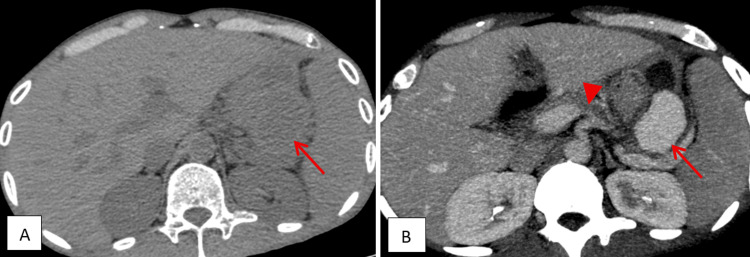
Axial sections of the non-enhanced (A) and venous phase (B) contrast-enhanced CT abdomen show a splenic vein varix within the pancreatic pseudocyst, arising from the splenic vein (red arrow). Narrowing of the splenic vein is seen posterior to the body of the pancreas (red arrowhead).
